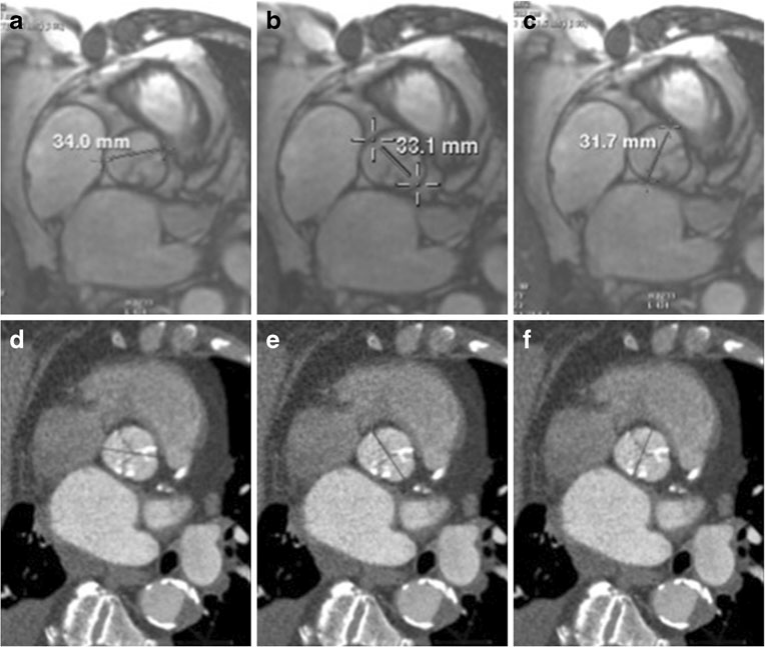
Diameters of sinuses of valsalva measured on MRI. 2-D SSFP of the aortic root in late diastolic phase is identified from the aortic root stack. Diameters are measured in mid-sinus above the aortic valve (a: noncoronary, b: left and c right). In the same patient corresponding CT images (d: noncoronary, e: left coronary and f: right coronary). Navigator-assisted 3-D SSFP or T2 black blood sequences can also be used for these measurements
